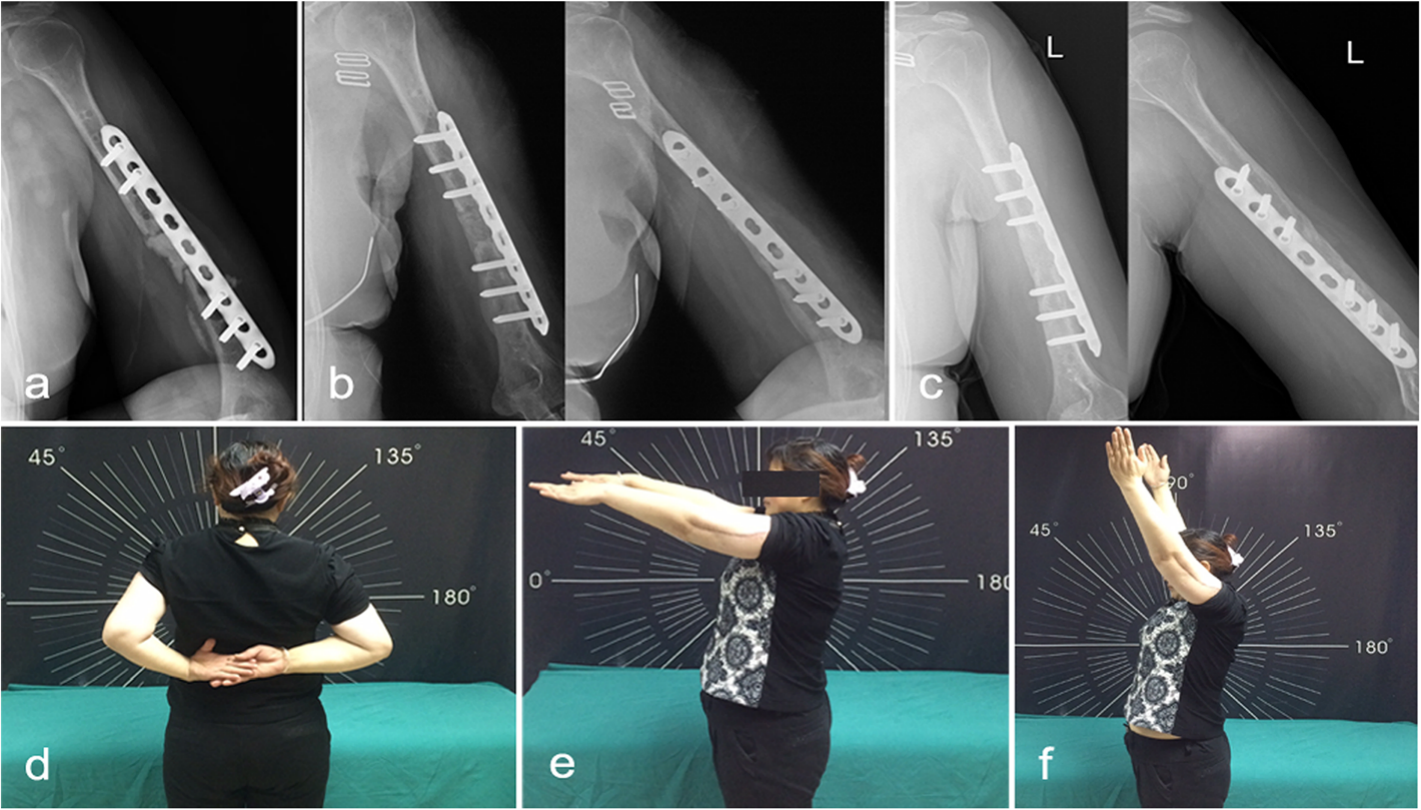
Treatment and follow-up results from a 45-year-old female with type humeral osteomyelitis (Patient 22). a AP radiograph shows a 2.8-cm defect after debridement with an antibiotic-coated cement and plate fixation in the first stage. b X-ray follow-up 4 months after bone grafting with clinical bone healing. c X-ray follow-up after 2 years with bone consolidation. d–f. Functional result at the final follow-up with good rotation (d), good flexion (e-f)
